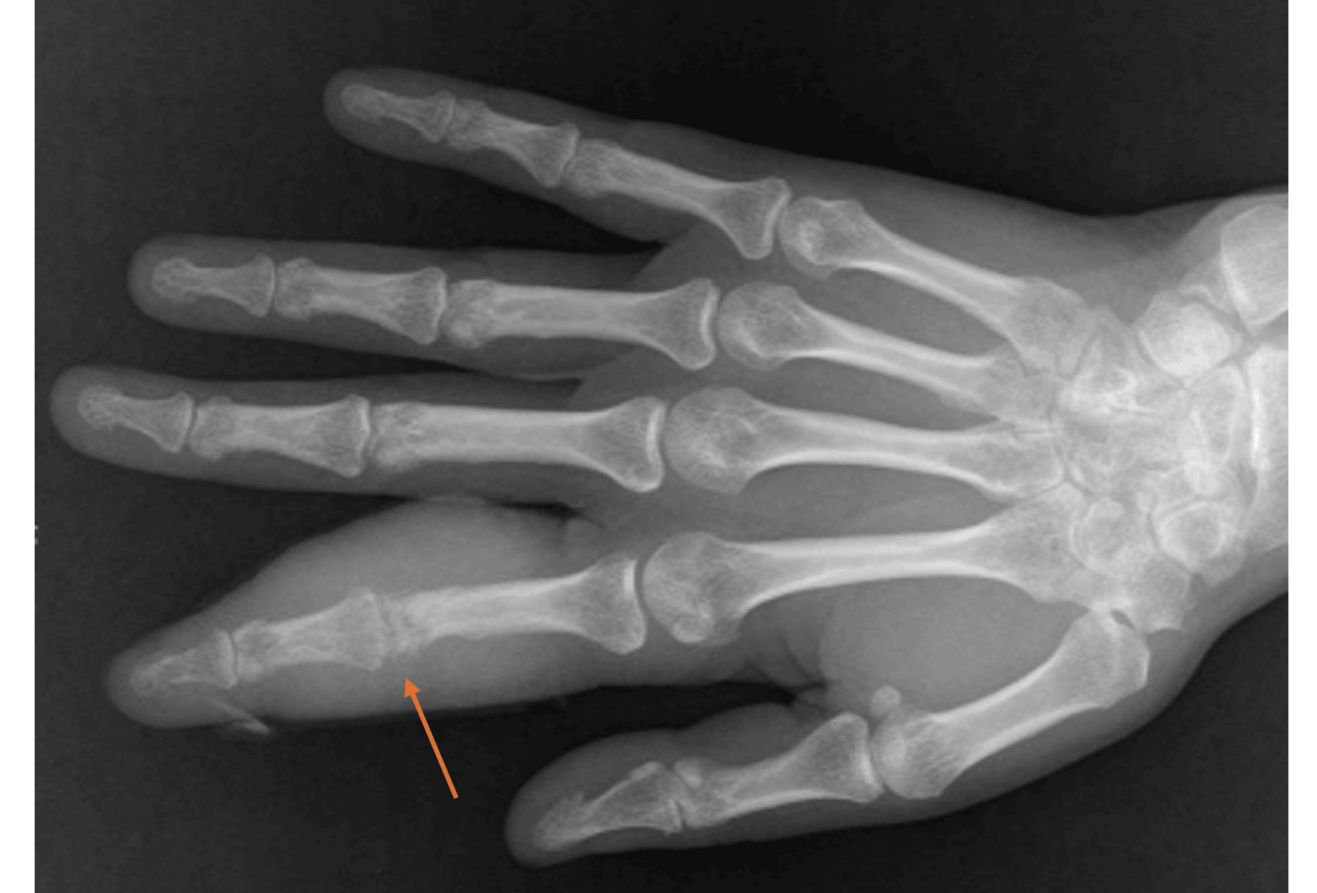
X-ray of the right hand. The arrow pointing to the tumor-associated erosion of the proximal interphalangeal joint of the second finger.
